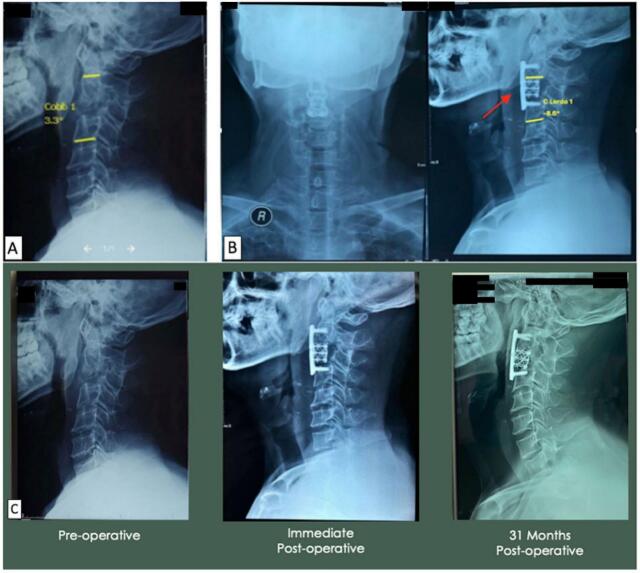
(A) Pre-operative lateral cervical X-ray; (B) Post-operative anteroposterior and lateral cervical X-ray using ACCF with mesh cage device (red arrow); (C) Consecutive lateral cervical X-ray from immediate post-operative to 31 months post-operative. Cervical fusion is observable, the fusion process on vertebrae cervical 3 using ACCF with mesh cage device remained intact and no failure was observed (red arrow). (For interpretation of the references to color in this figure legend, the reader is referred to the web version of this article.)
